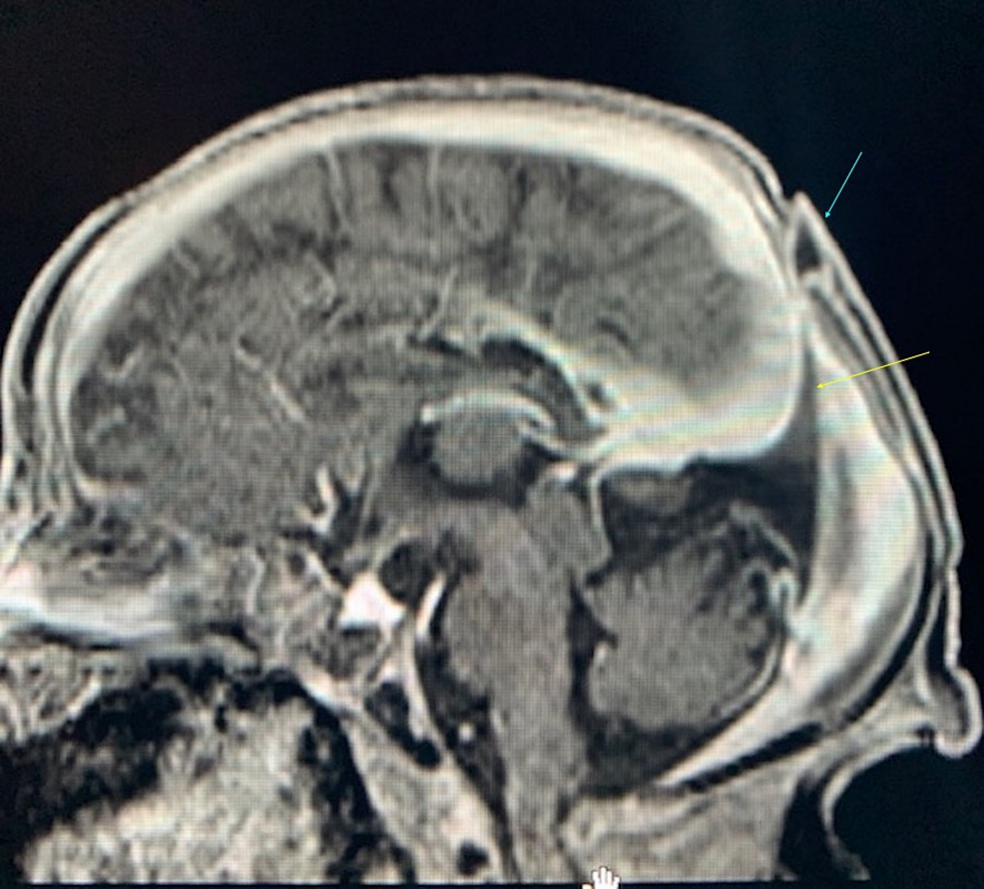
Sagittal FLAIR post-contrast MRI. The image is showing lack of enhancement at the level of scalp lesion (blue arrow), indicating a fibrous rather than venous substance, contrasting with sinus pericranii. Beaked tentorium is also shown again (yellow arrow). FLAIR: fluid-attenuated inversion recovery
